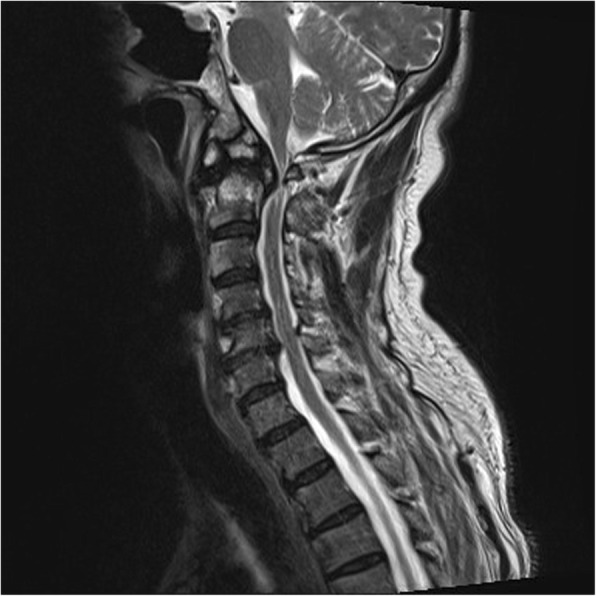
MRI image of hypertrophic ossification at the odontoid process, tight stenosis at the C1, and edematous changes at the spinal cord
